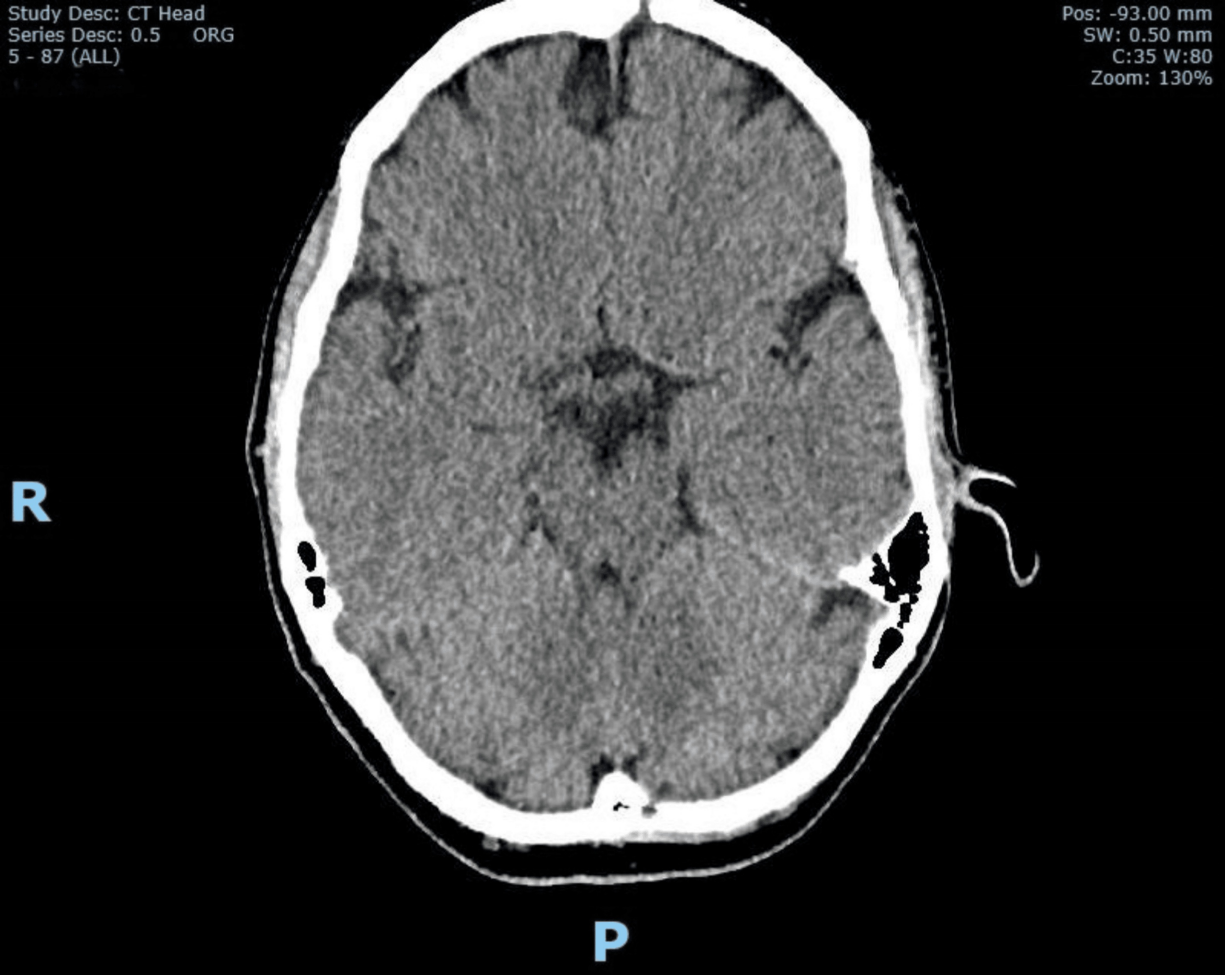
Normal unenhanced CT brain CT: computed tomography
